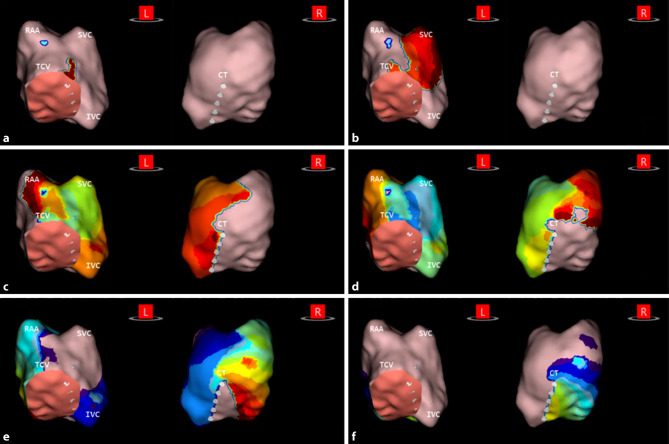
Example of right-sided atypical flutter in patient 16. Propagation-history map of right atrium, which uses bands of colour to show location and velocity of leading edge of wave front over a set period of time. Red colour indicates leading edge of wave front, with trailing colour bands showing earlier locations. Width of colour bands indicates conduction velocity of wave fronts; wider bands are indicative of fast conduction and narrow bands of slow conduction. a Focal activation at tricuspid annulus. b Propagation of wavefront towards superior vena cava (*SVC*). c Separation into two wavefronts towards inferior septum and lateral wall, respectively. d Propagation of two wavefronts down septum and lateral wall. e, f Collision of two wavefronts at cavotricuspid isthmus. *L* left, *R* right, *RAA* right atrial appendage, *TCV* tricuspid valve,* IVC* inferior vena cava, *CT* crista terminalis
